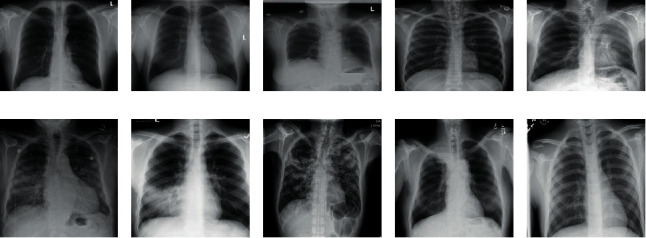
Chest radiographs of patients used for the assessment of competence. The expected diagnoses in line with the specialists' interpretations were (a) pneumothorax, (b) normal image, (c) pneumoperitoneum, (d) pneumomediastinum/left rib fracture, (e) lung collapse, (f) pulmonary edema/congestive cardiac failure, (g) pneumonia, (h) tuberculosis/pneumoconiosis/metastasis, (i) Pancoast tumour/*tuberculosis*, and (j) clavicular fracture/acromioclavicular dislocation.
